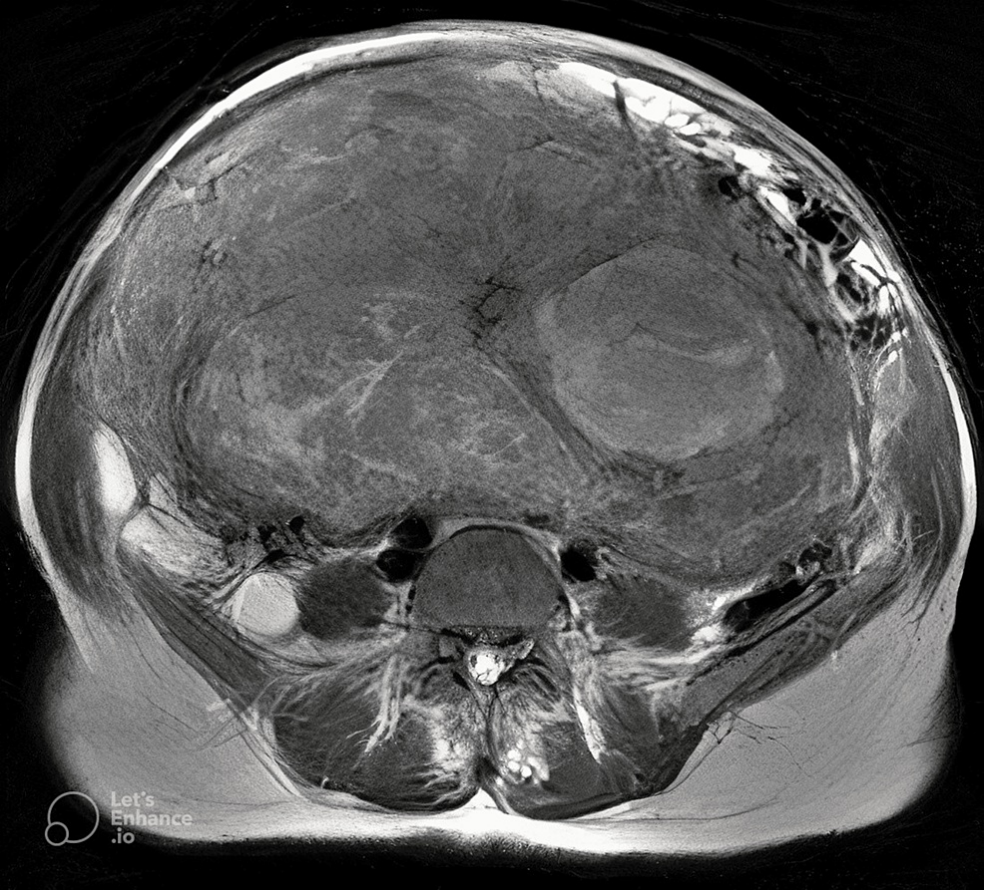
Axial T2-weighted image showing a grossly enlarged uterus with multiple T2 heterogenous signal intensity intramural fibroids in the lower uterine segment and extensively thickened myometrium in the fundal region and upper uterine segment (1.5 Tesla MRI).
